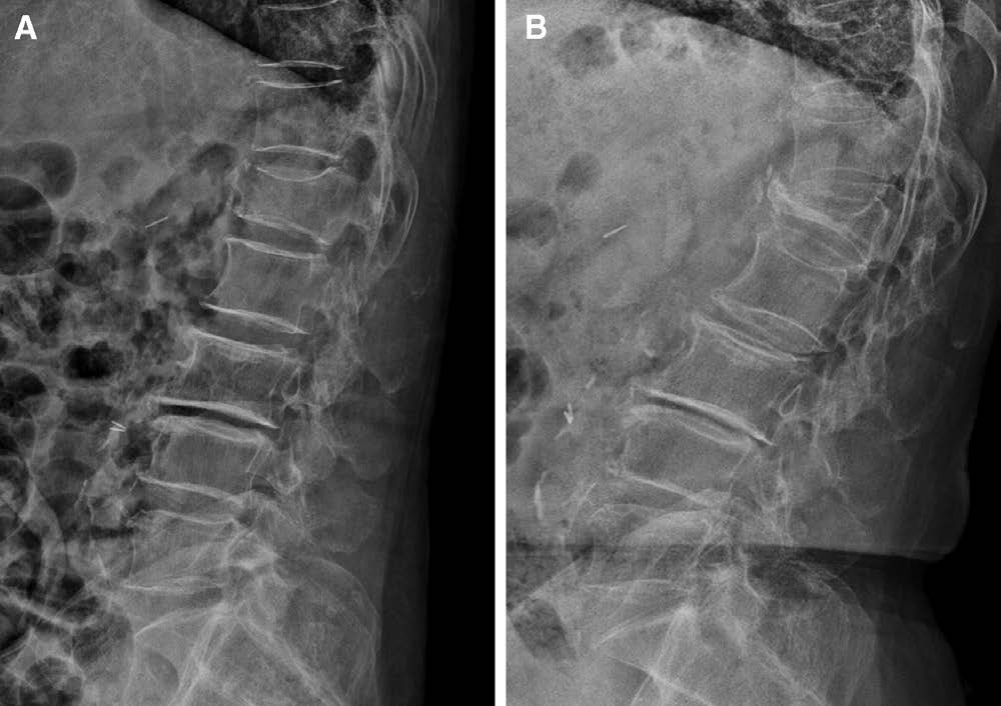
The case study of a 77-yr-old female patient with an L1 compression fracture treated with teriparatide. (A) The pretreatment lateral radiograph showing a VCR of 7.34% and a CA of 21.5º. The pretreatment BTM values were as follows: OC (4.38 ng/mL), serum CTX (0.21 ng/mL), vitamin D (11.23 ng/mL), and PTH (67 pg/mL). (B) The posttreatment lateral radiograph showing a VCR and CA progression of 65.15% and 24º, respectively, after 3.5 mo. The posttreatment BTM values were as follows: OC (29.18 ng/mL), serum CTX (0.42 ng/mL), vitamin D (24.19 ng/mL), and PTH (34 pg/mL). BTM = bone turnover markers, CA = Cobb angle, CTX = C-terminal telopeptide of collagen, OC = osteocalcin, PTH = parathyroid hormone, VCR = vertebral compression ratio.
